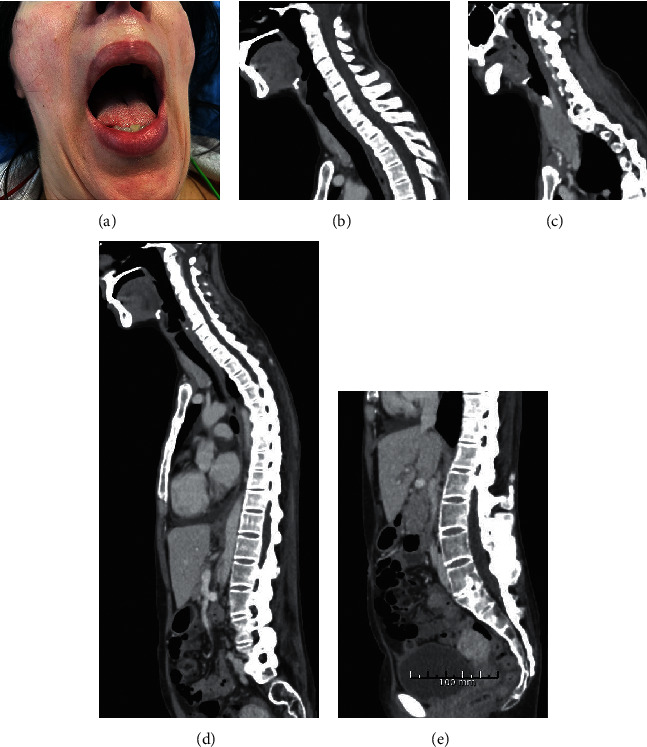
The patient demonstrating mouth opening of 25 mm (a). CT scan of the cervical column showing the midline view with block vertebrae C2/C3 and C5/C6 (b) and paramedian view showing fusion of facet joints C2–C6 (c). Ossification of the anterior longitudinal ligament with anterior bridging osteophytes seen in the thoracic region (d) and fusion of all spinous processes in the lumbar region (e).
